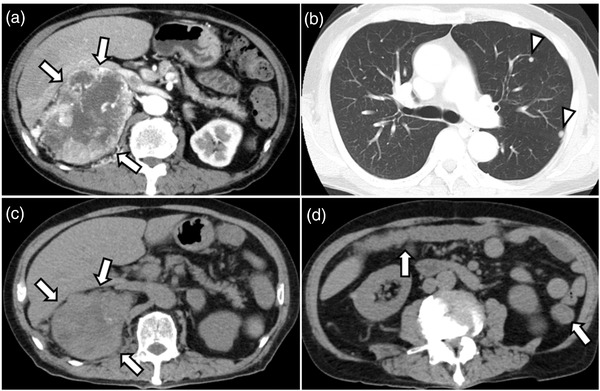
Computed tomography (CT) findings. (a, b) First abdominal CT shows a 15 cm tumor in the right kidney with heterogeneous contrast in the arterial phase (arrows) with multiple lung metastases (arrowheads). (c) After three courses of immune checkpoint inhibitor therapy, CT showed that the primary tumor had decreased in size (arrows). (d) Three days after the end of the third course, CT shows wall thickening throughout the colon (arrows)
